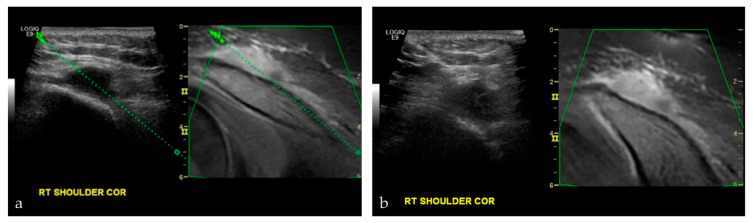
A 17-year-old male with nasopharyngeal carcinoma. (a,b) were taken during an US-MRI guided fusion biopsy of a soft tissue mass to the right shoulder, just superior to the acromioclavicular joint. Final biopsy results revealed metastatic disease from nasopharyngeal carcinoma.
